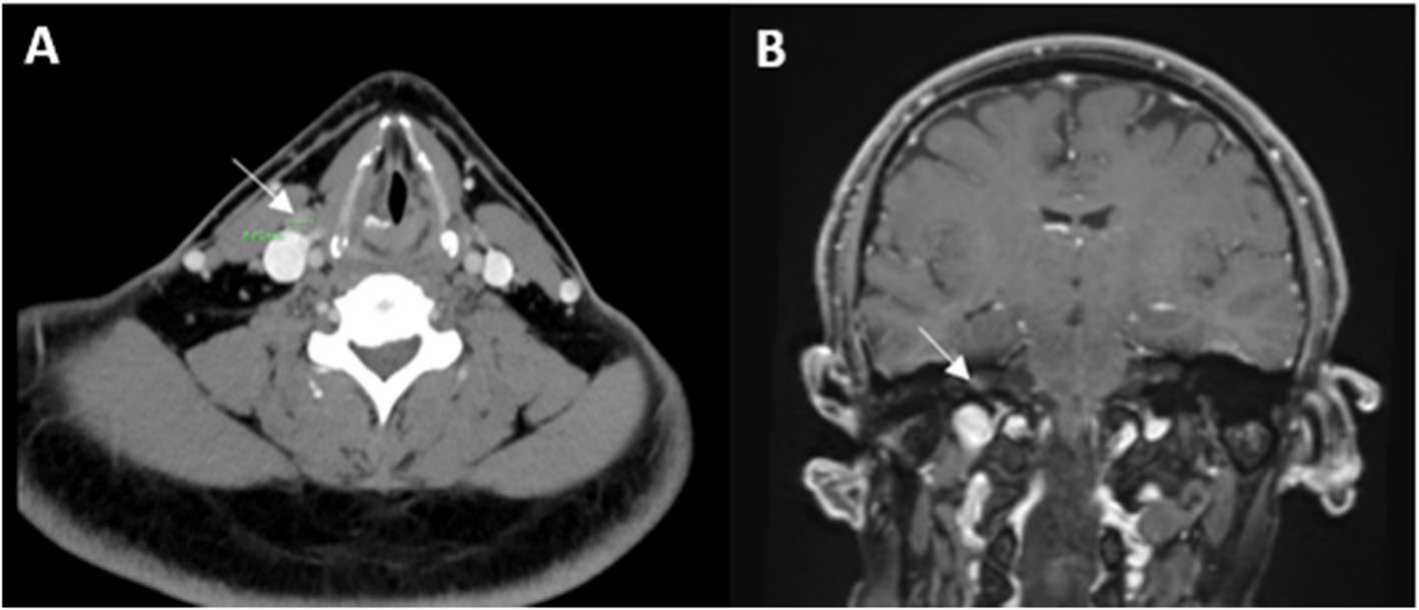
A Axial neck CT image demonstrating a 1 cm right supraclavicular reactive lymph node (arrow). B Coronial brain (with Gadolinium) MRI image showing enhancement in the right auditory canal, suggesting involvement of Cranial nerves VII and VIII (arrow)
